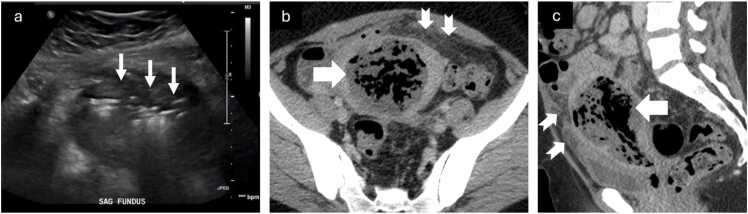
30-year-old female post caesarian section day 21 with postpartum haemorrhage, fever and ongoing purulent vaginal discharge. (a) Transabdominal ultrasound displays bulky uterus with multiple air foci in the endometrial cavity with distal shadowing and ring-down artifact (thin arrows). Contrast enhanced CT pelvis (b) axial and (c) sagittal images show gas and fluid distended endometrial cavity (bold arrows) with parametrial inflammation and fat stranding extending into the pro peritoneal fat (notched arrows). DIAGNOSIS: Endometritis.
